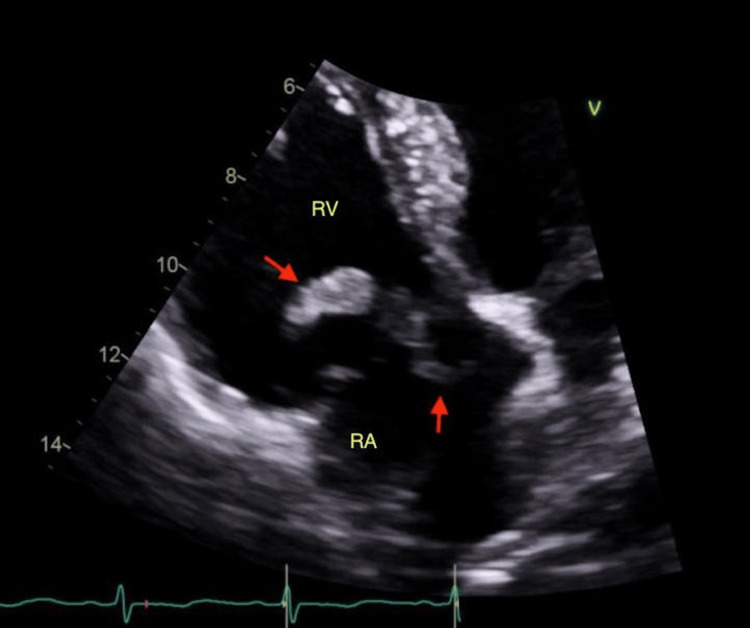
Transthoracic echocardiogram of the clot in transit Apical four-chamber view: this image reveals thickened tricuspid leaflets with two mobile echo densities seen on the atrial and ventricular valve surfaces (indicated with arrows). RA: right atrium; RV: right ventricle
